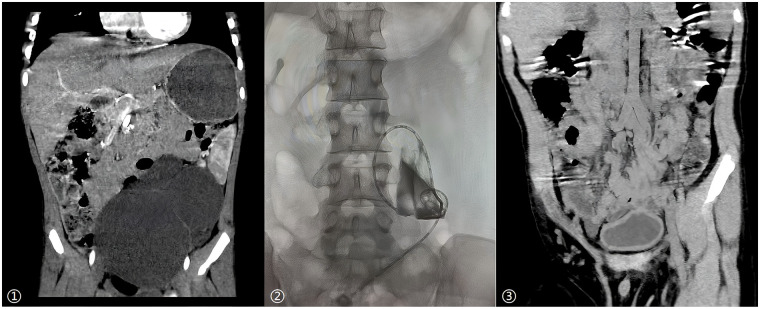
(1) preoperative coronal CT image showing a large cystic lesion in the pelvis. (2) Intraprocedural image obtained during interventional drainage: after percutaneous puncture, a drainage catheter was placed and contrast agent was injected, opacifying the cyst cavity. (3) Postoperative 8-week follow-up coronal CT image demonstrates significant interval reduction of the cystic lesion.
